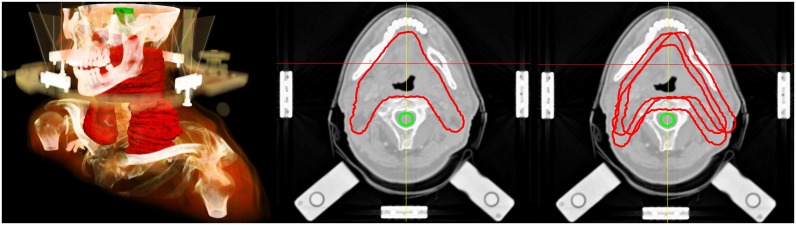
Clinical Target Volume (CTV) of a head and neck cancer patient. Left: Volume rendering of the planning CT image for an exemplary head and neck cancer patient receiving an IMRT treatment under daily image guidance. The clinical target volume (CTV) is displayed in red, brainstem in green. The stereotactic external frame is used as positioning device. Center: A transversal CT slice of the same patient of the mandibular region is displayed. The clinical target volume (CTV, red) and the spinal cord (green) as organ at risk are shown as planning contours. Right: CTV contours of different treatment days are plotted on the same planning CT slice to visualize daily variations.
